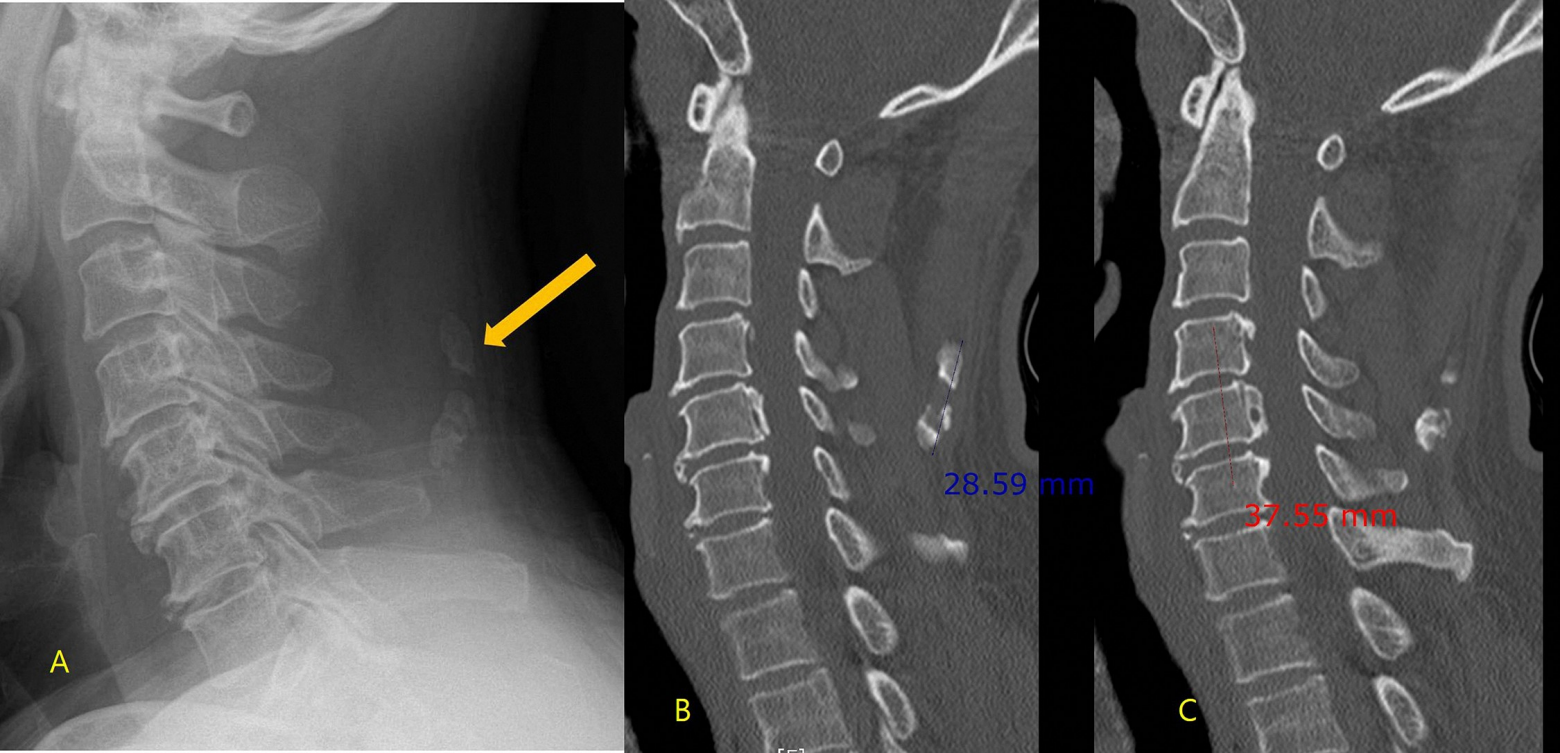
60-year-old man with neck discomfort and mobility disturbance after trauma. (A) Lateral radiograph of the cervical spine showing ossification of the nuchal ligament (arrow). However, ossification of the posterior longitudinal ligament was not clearly visible. (B) The sagittal reconstructed CT image revealed ossification of the nuchal ligament extending from C4 to C5 and measuring 2.9cm along its entire length. (C) Another CT slice showing ossification of the posterior longitudinal ligament extending from C4 to C6 and measuring 3.8cm along its entire length.
